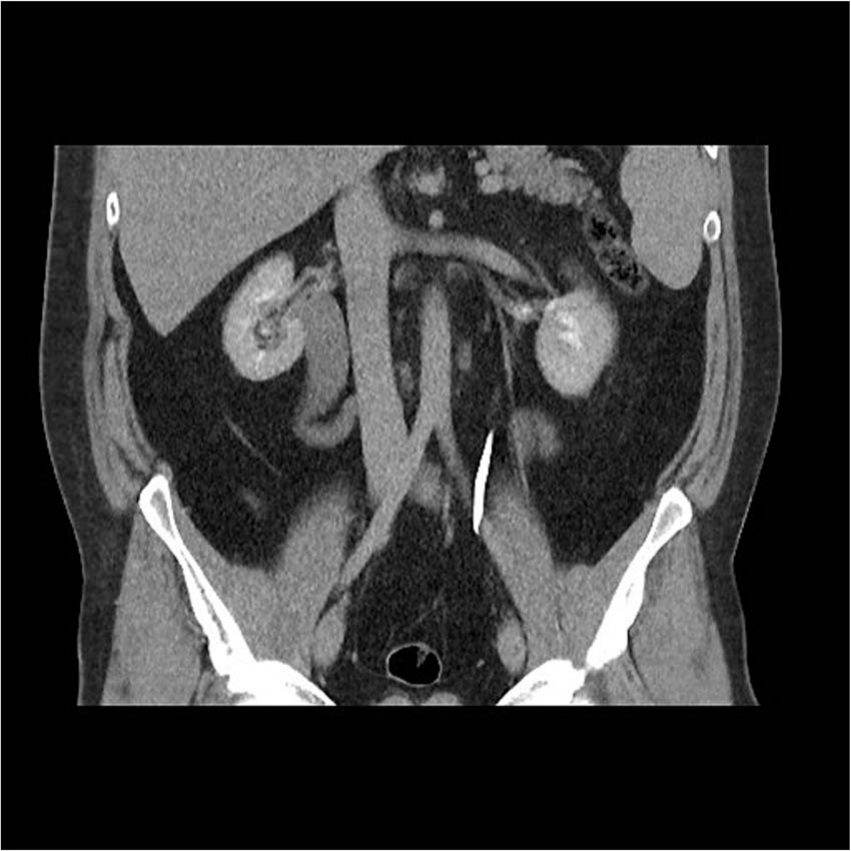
CT abdomen shows an S-shaped ureter with the right ureter passing behind the IVC.
